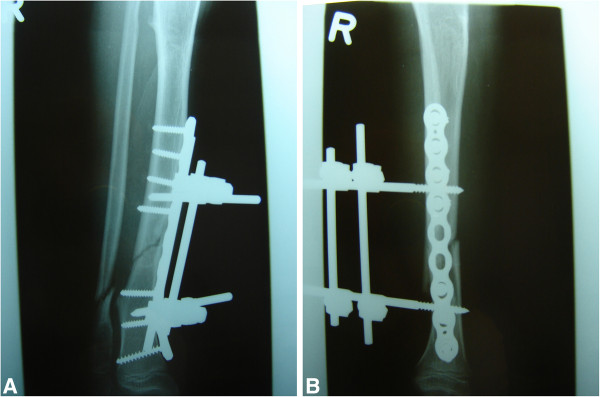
(a,b) Post-operative plain radiograph of the lower extremity after osteosynthesis and application of an external fixateur (anterior-posterior and axial).
